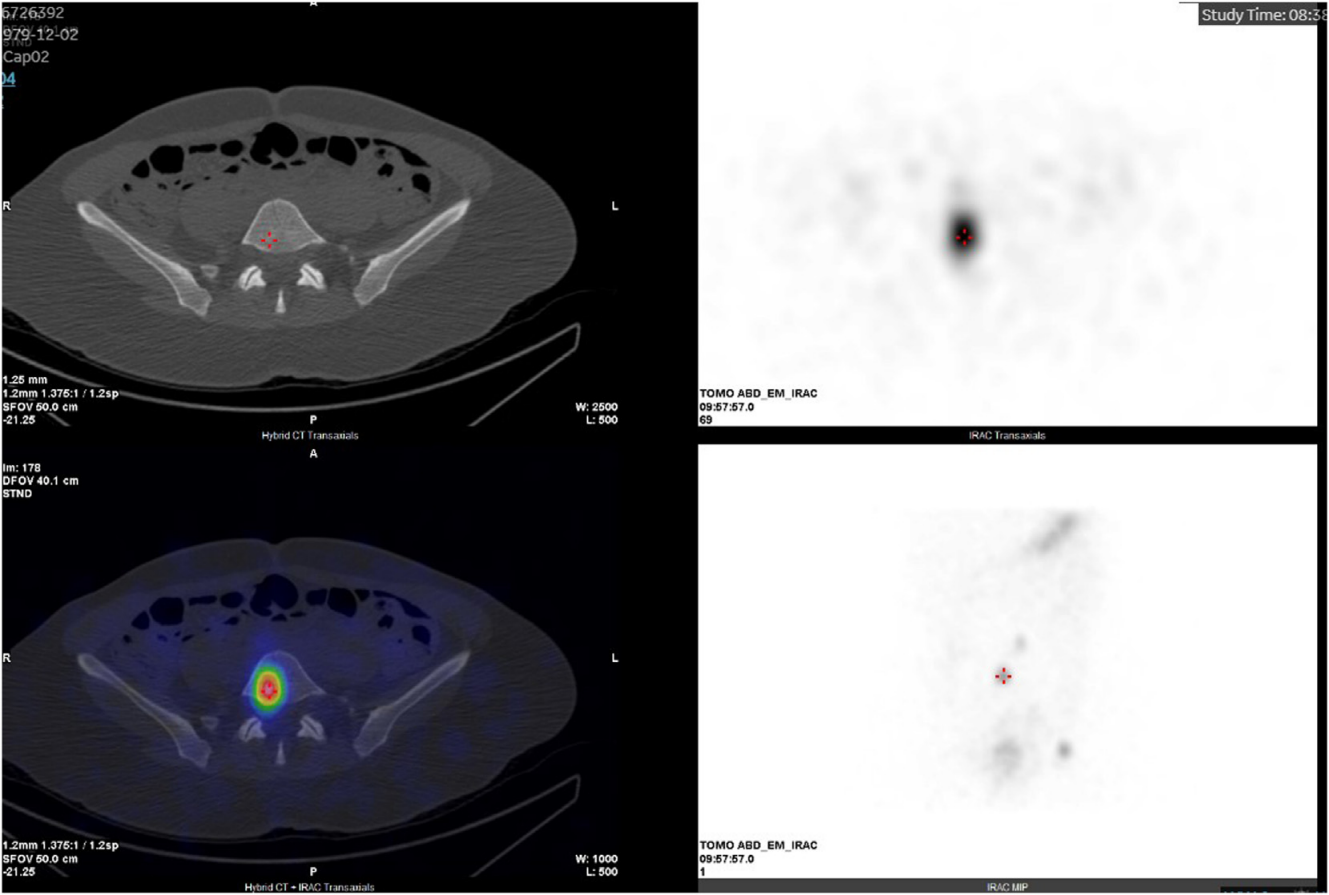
SPECT/CT and WBS (with overlap) showing nonmetastatic radioactive iodine uptake in the L5 vertebra. *WBS*, whole-body scan.
